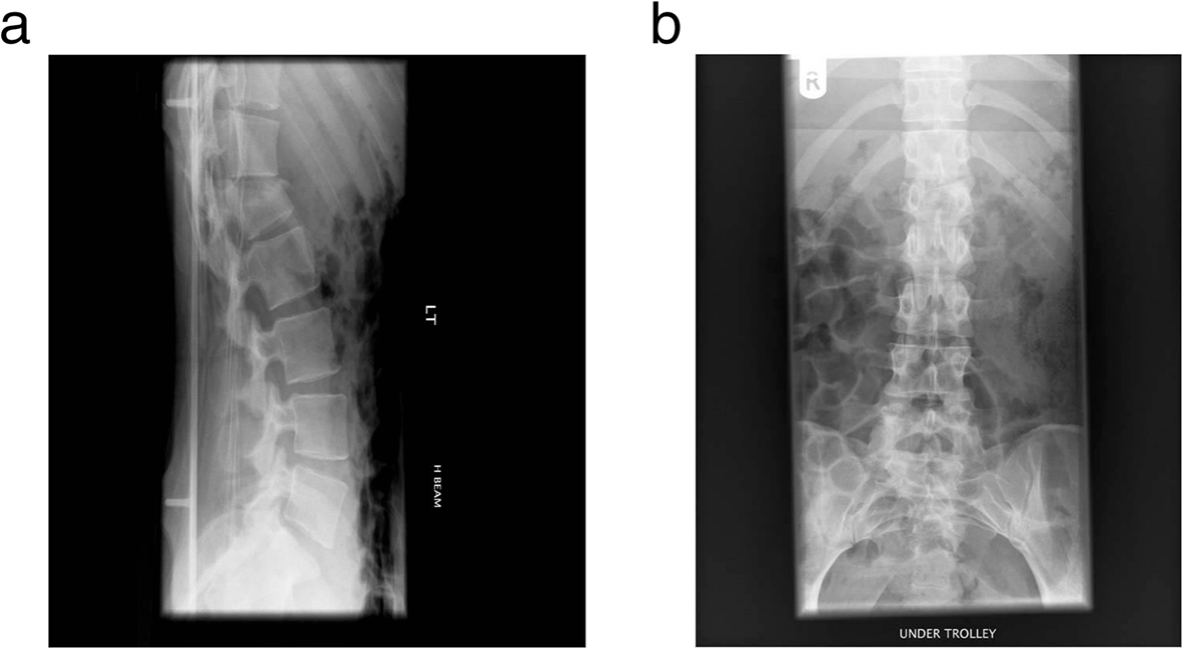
Radiograph showing a single level L1 fracture at time of injury a. Lateral b. Antero-posterior.
